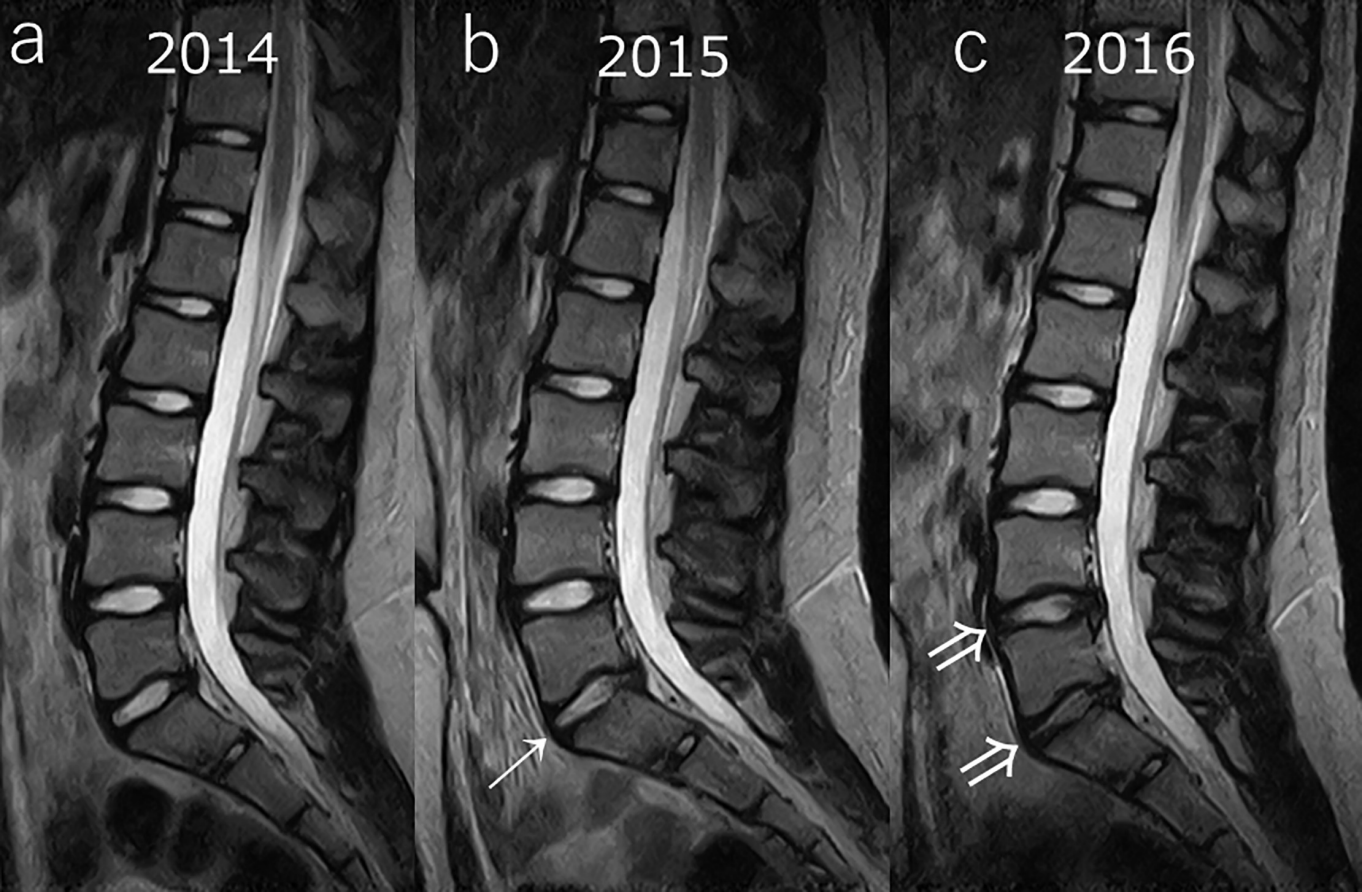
Representative case #2 MRI of a 12 year-old girl at the start of the study in 2014. There were no findings in 2014 (a); however, lumbar disc protrusion and disc degeneration were noted at the L5/S1 level in 2015 (b: →), and at the L4/5 and L5/S1 levels in 2016 (c: ⇒). The degenerative changes were irreversible at this point.
